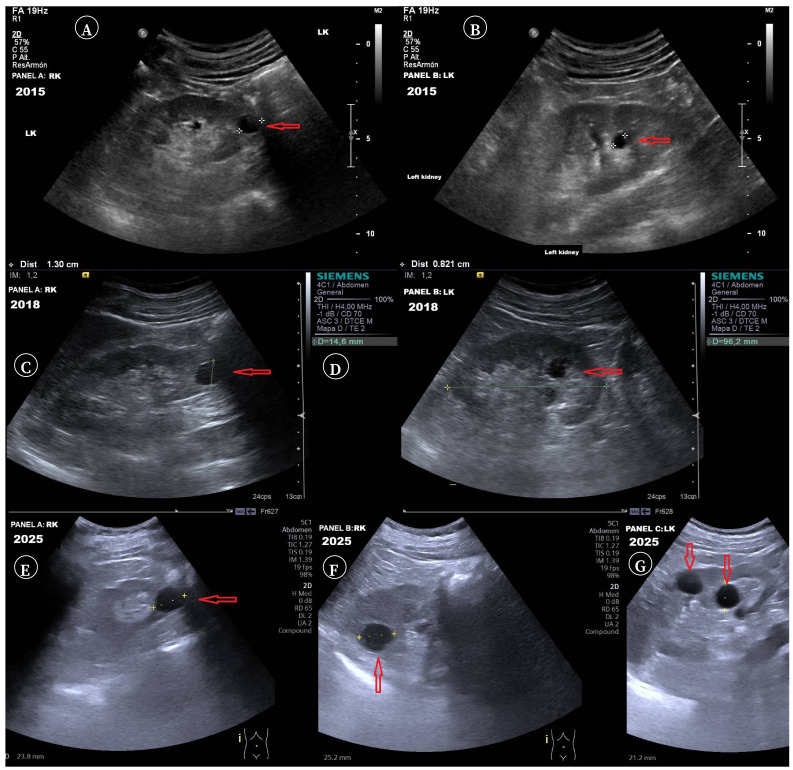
Changes observed in the urinary system during the period 2015-2025 by ultrasound. A, B. Small bilateral cortical cysts and sinus cysts in the left kidney (2015). C, D. 2018. Bilateral cortical cysts, the largest measuring 15 mm in the lower pole of the right kidney (C) and left sinus cysts measuring up to 16 mm (D). E, F. 2025. Right kidney: cortical cysts measuring 21 to 25 mm. G. 2025. Left kidney sinus cyst measuring 21 mm and cortical cyst measuring 28 mm. Red arrows: location of the renal cysts.
